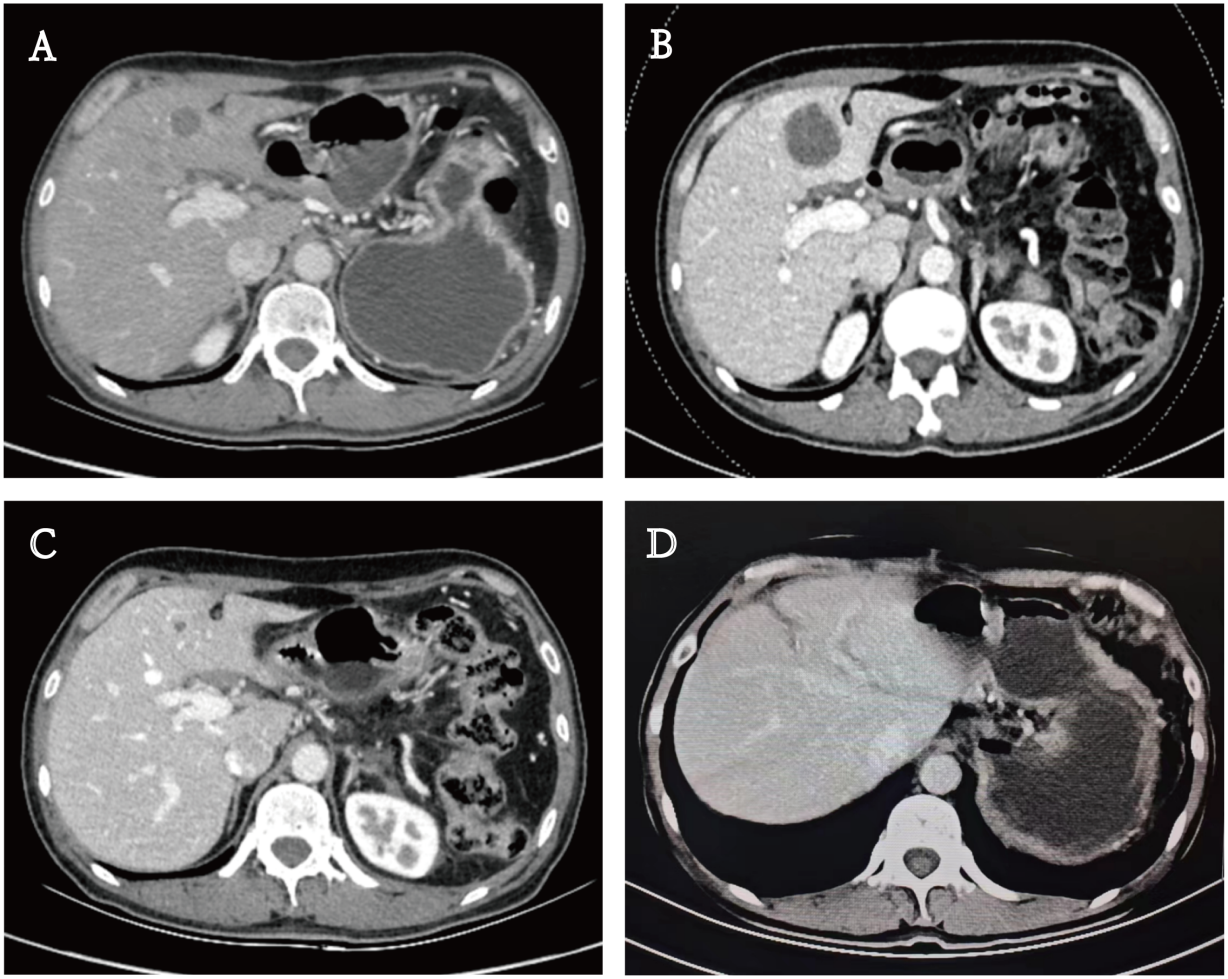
Enhanced computed tomography (CT) scan. (A) A low-density lesion in S4 of the liver. (B, C) Lesion size changes in the treatment process. (D) Imaging at three months postoperatively.
